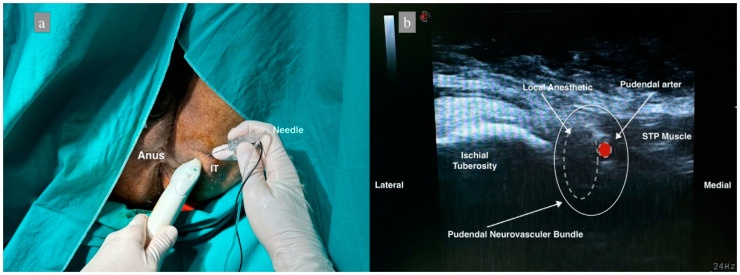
Patient position and sonoanatomy during ultrasound-guided pudendal nerve block. (a) Patient in lithotomy position, IT: Ischial Tuberosity. (b) Dashed white lines represent the distribution of local anesthetic, dashed red figure shows the pudendal artery, STP: Superficial transverse perineal muscle.
